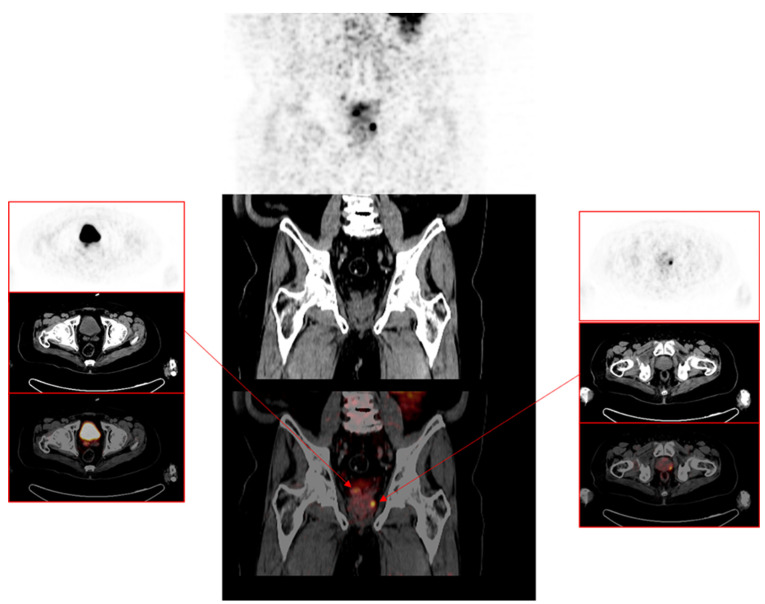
A 77-year-old male previously treated with external beam radiotherapy for adenocarcinoma of the prostate, Gleason 4+4. ^68^Ga-PSMA-11 PET/CT was requested for the localization of disease recurrence at serum PSA of 0.61 ng/mL. Central images are the coronal PET-only, CT-only, and fused PET/CT images of the pelvis, localizing disease recurrence to the right seminal vesicle (right insert) and a focal lesion in the left lobe of the prostate gland (left insert).
